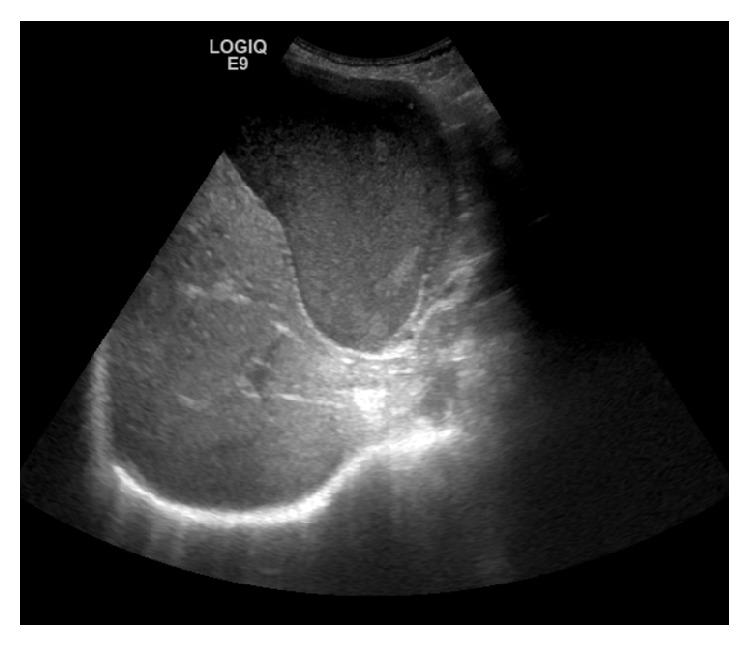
Ultrasound abdomen demonstrating a grossly distended gallbladder with a thickened posterior wall.
